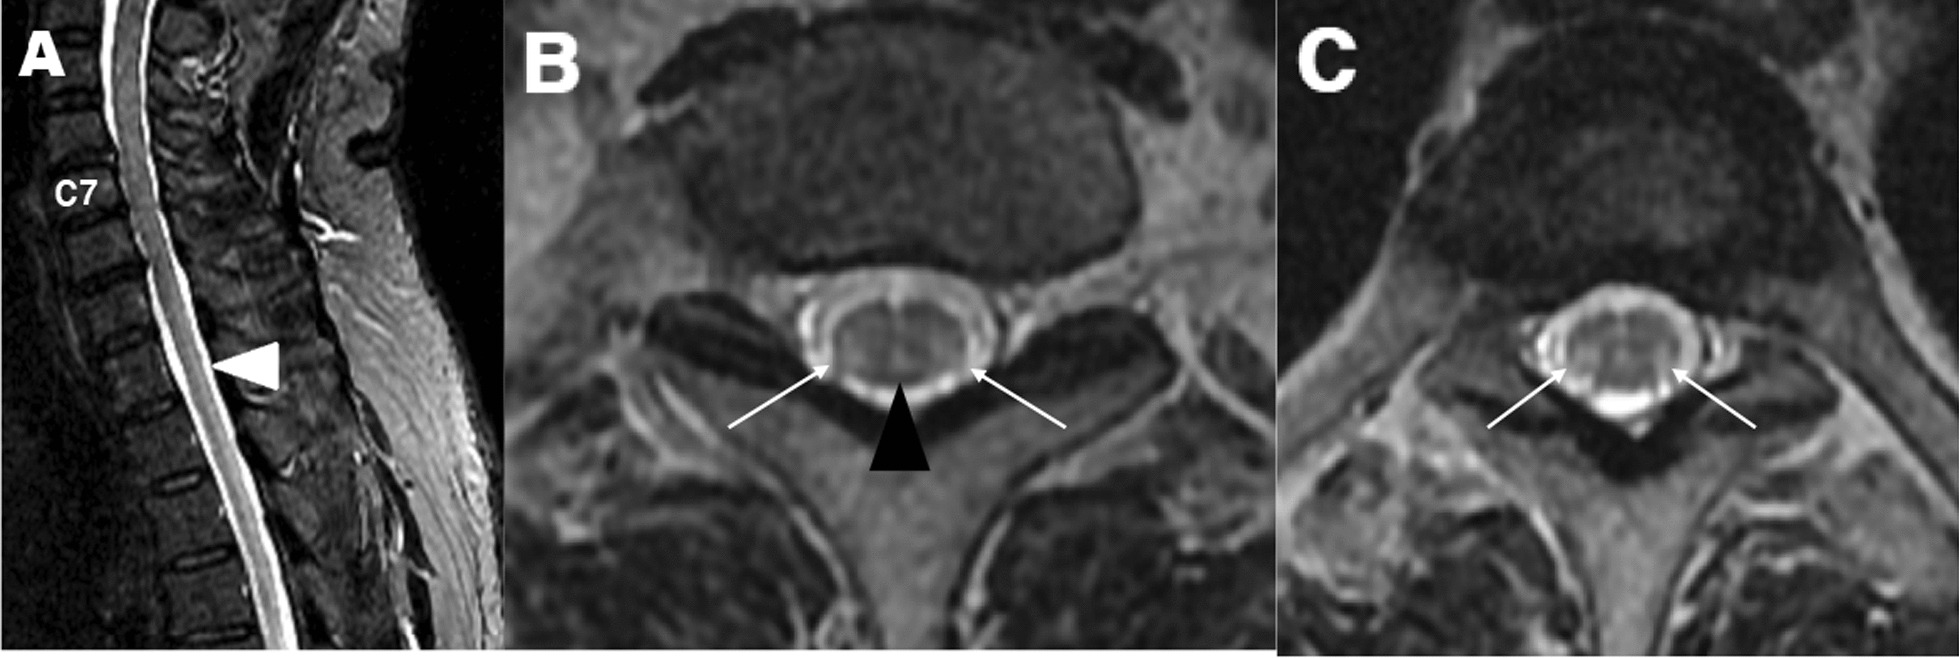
Spinal cord MRI of the patient. Sagittal T2 images showed longitudinal T2 hyperintensity of the posterior columns at upper thoracic level (white arrowhead) (A) and axial T2 images confirmed bilateral, symmetric high signal intensities of lateral corticospinal tracts (B, C, white arrows) and the gracile tracts (B, black arrowhead) at upper thoracic level without gadolinium enhancement
